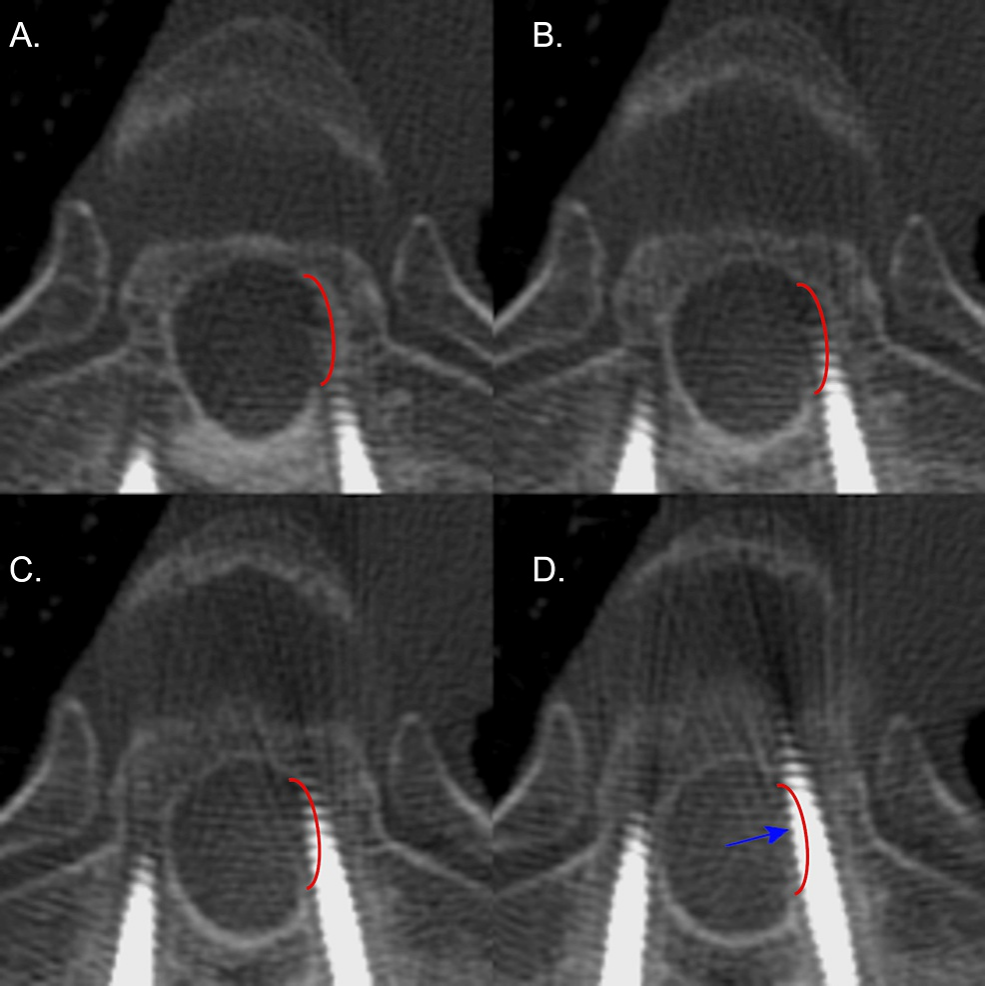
Axial CT radiographs of the left T6 pedicle screw on patient 5. Axial CT radiographs demonstrating the left T6 pedicle screw placement on patient 5 with a medial breach. Screw placement progresses from A to D. The pedicle of interest is outlined in red. The blue arrow denotes the largest deviation of the screw. Please note all CT images are in radiographic orientation.
